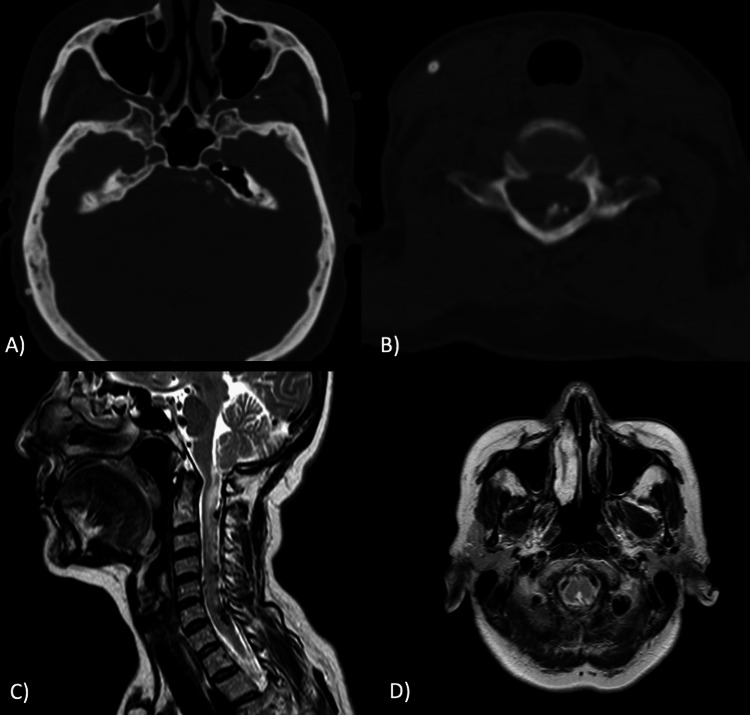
A) Axial non-contrast CT scan of the brainstem with amorphic arachnoid calcifications extending from the intracranial cisterns with cervical extension from C1 to C7 (B) with posterior predominance. C) Weighted (sagittal) and D) T2-weighted axial MRI with increased T2 hyperintense signal change within the cord with compressive myelopathy.
